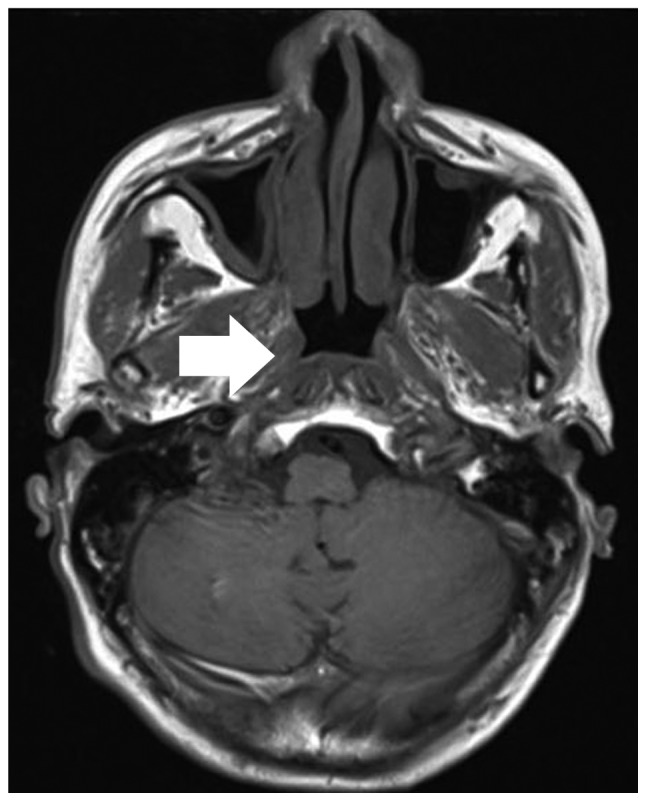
Magentic resonance imaging (MRI) at 11 months post anti-tumor therapy (July, 2012) revealing no evident thickening of the nasopharyngeal wall (arrow).
